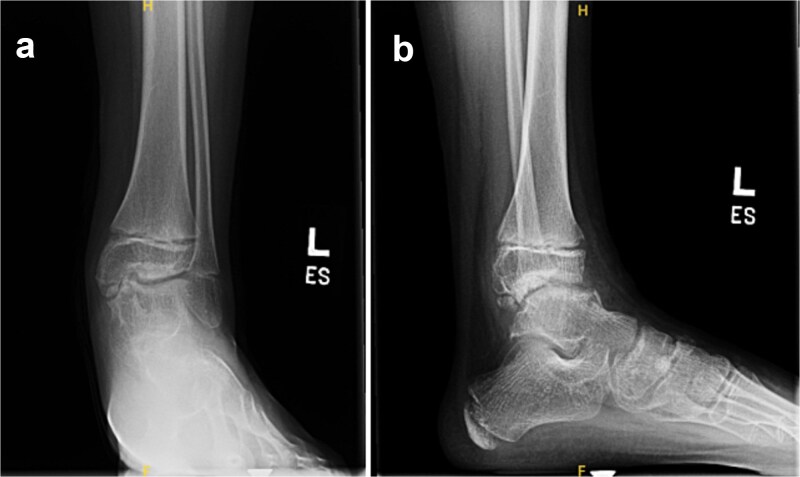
Postoperative AP (a) and lateral (b) radiographs of the left ankle demonstrating persistent irregularity of the distal tibia and talus with residual osteochondral changes following the third excision.
